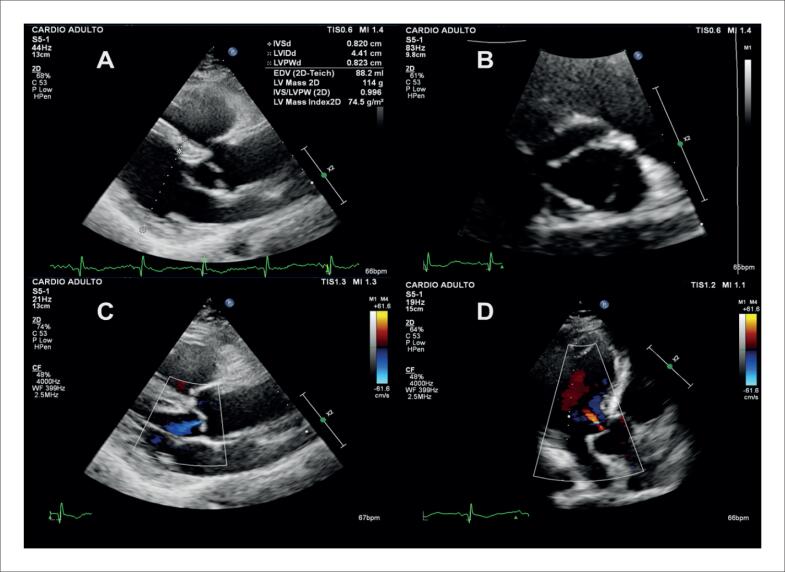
Paciente com diagnóstico de artrite reumatoide apresenta, ao ecocardiograma transtorácico, espessamento discreto valvar aórtico ao corte paraesternal longitudinal (A) e transverso (B) com refluxo de grau discreto ao Doppler colorido (C) e (D).
